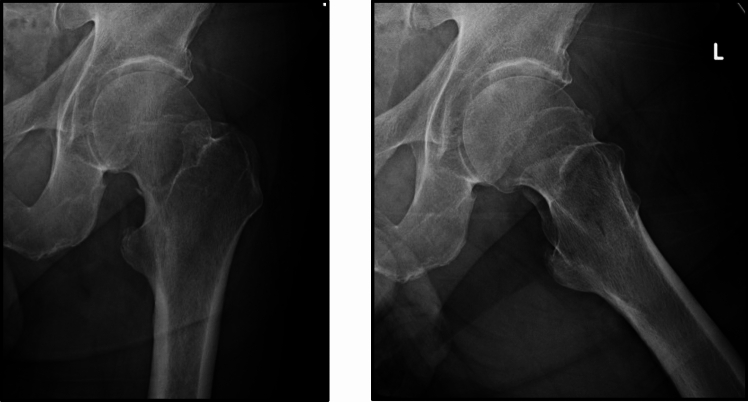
Radiographs of a non-displaced femoral neck fracture
